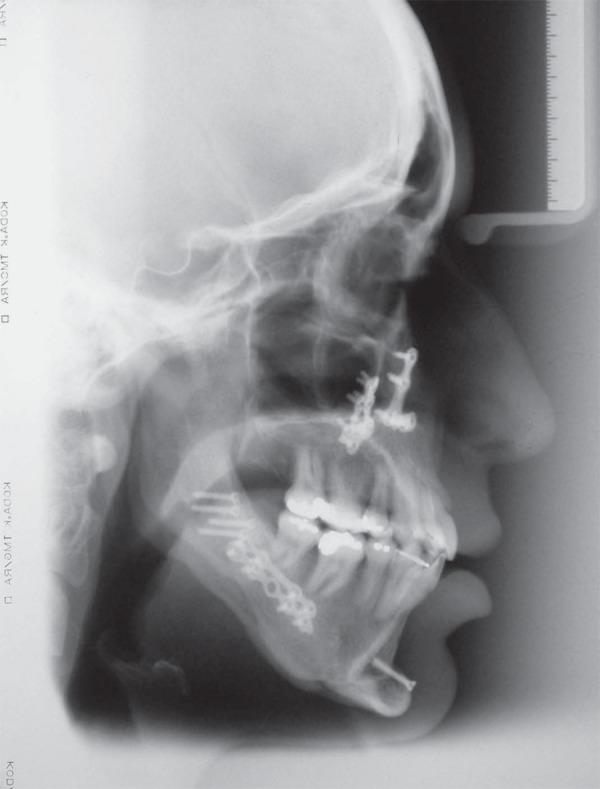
Fourteen-month cephalometric radiograph showing occlusion plane changing and lower
